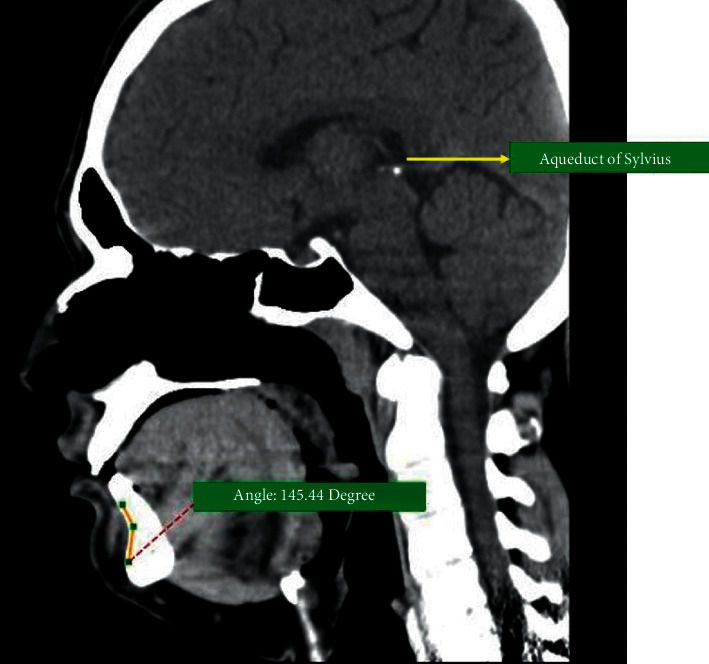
Chin angle in a 2D CT scan of a condylar fracture patient.
